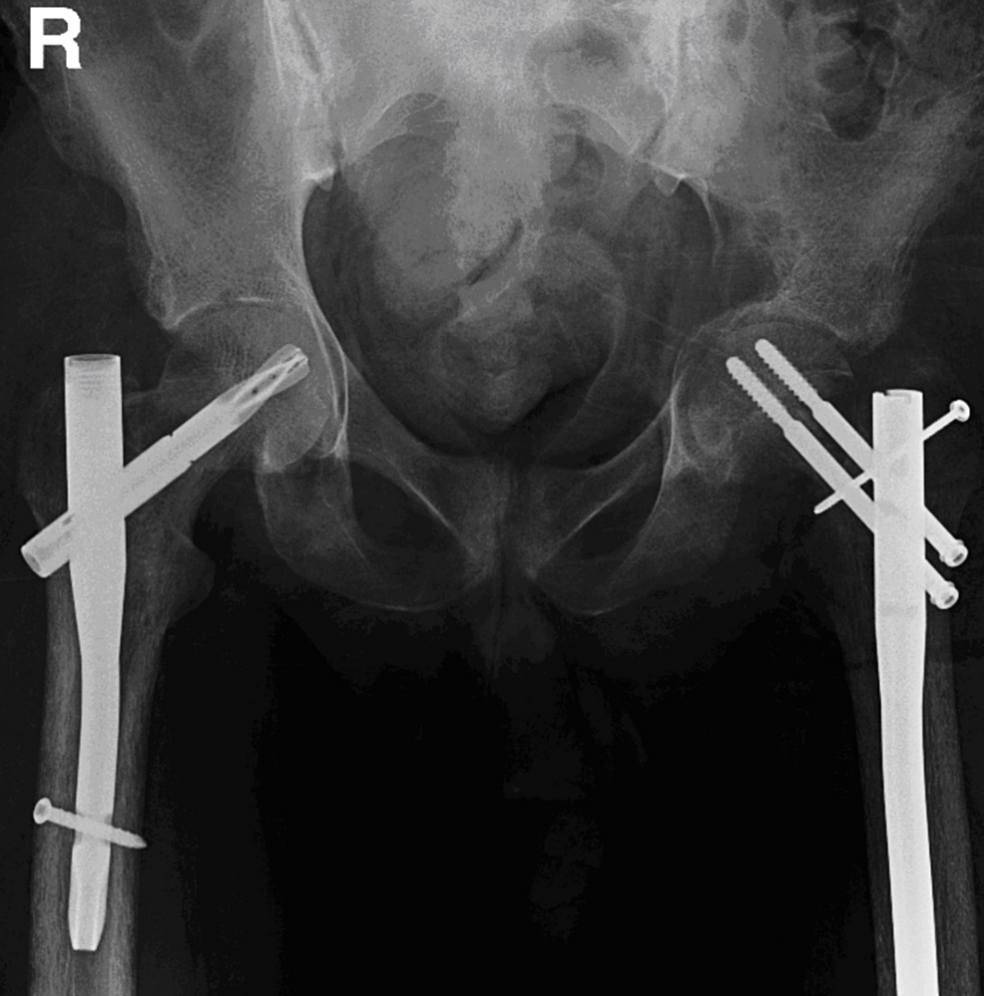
Plain radiograph of the pelvis: post left cephalomedullary nail and right proximal femoral nail insertion
